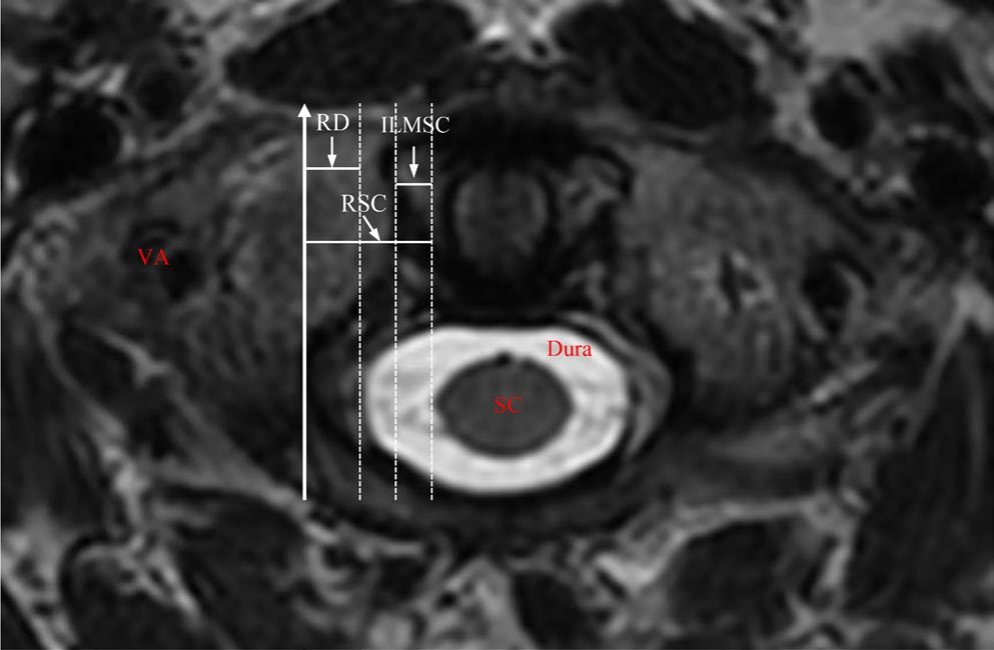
The axial plane of the atlas in MRI scan. The white solid line with arrow was the ideal trajectory of C1ZAS. The white‐dashed lines were parallel lines of the trajectory of C1ZAS. RD was the distance between the recess and dura. RSC was the distance between the recess and the SC. ILMSC was the distance between the inner of the lateral mass and the SC.
